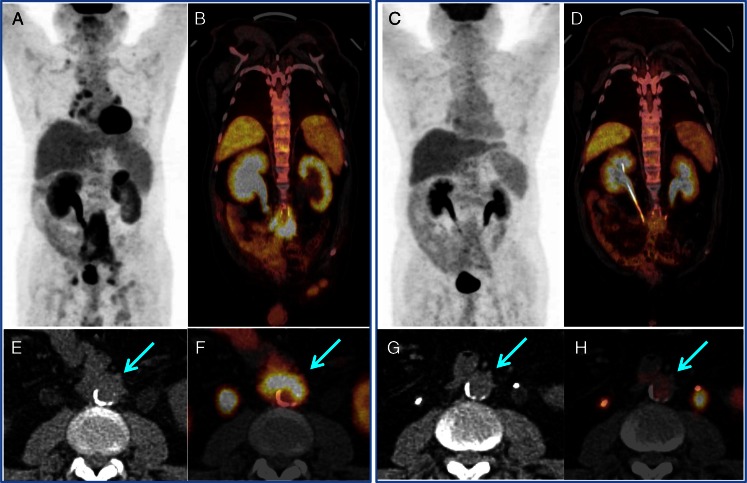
^18^F-FDG PET/CT guided the timely ureter recanalization in an IgG4-RD patient with retroperitoneal fibrosis and aorta involvement. The enlarged right renal pelvis with radioactive urine retention indicated severe ureteral obstruction, whereas the left side without radioactivity indicated complete obstruction (a and b). After D-J tube cannulation, the renal function was recovered bilaterally (c and d); the intense-uptake lesions were smaller and the intensity was significantly lower in response to the steroid treatment. The *arrows* show the aorta involvement beside the retroperitoneal fibrosis (e, f), which has a complete response to steroid-based treatment (g, h)
